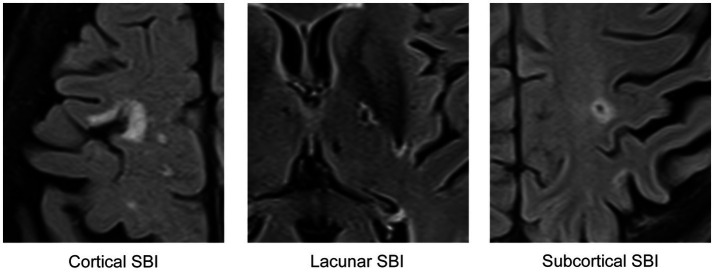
FLAIR/T2-weighted images displaying representative examples of the three distinct categories of brain infarcts utilized in this study. Each depicted infarct is chronic and was identified in asymptomatic patients, classifying them as SBIs.
